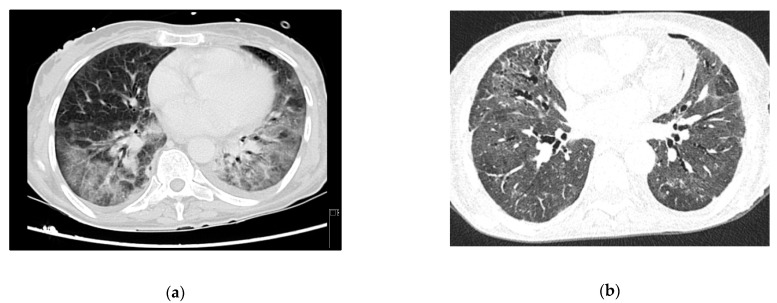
Case 3 thoracic CT-scan at diagnosis (a) and follow-up (b).
